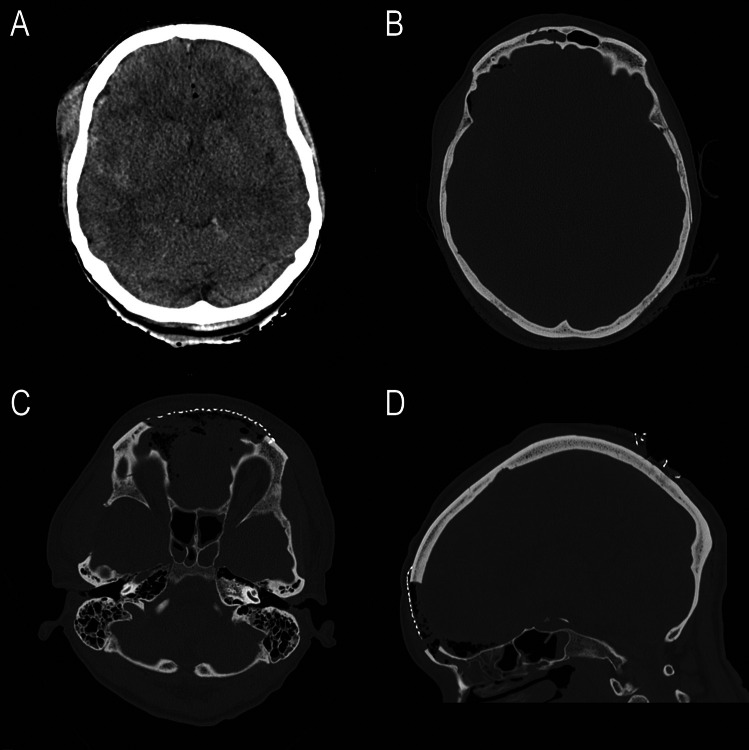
Patient 5: (A) initial post traumatic CT scan: brain swelling and small subdural hematoma on the right side, intracranial air (B) frontobasal fracture (C) and (D) postoperative CT scans, first postoperative day, frontal bone defect covered with a titanium mesh
